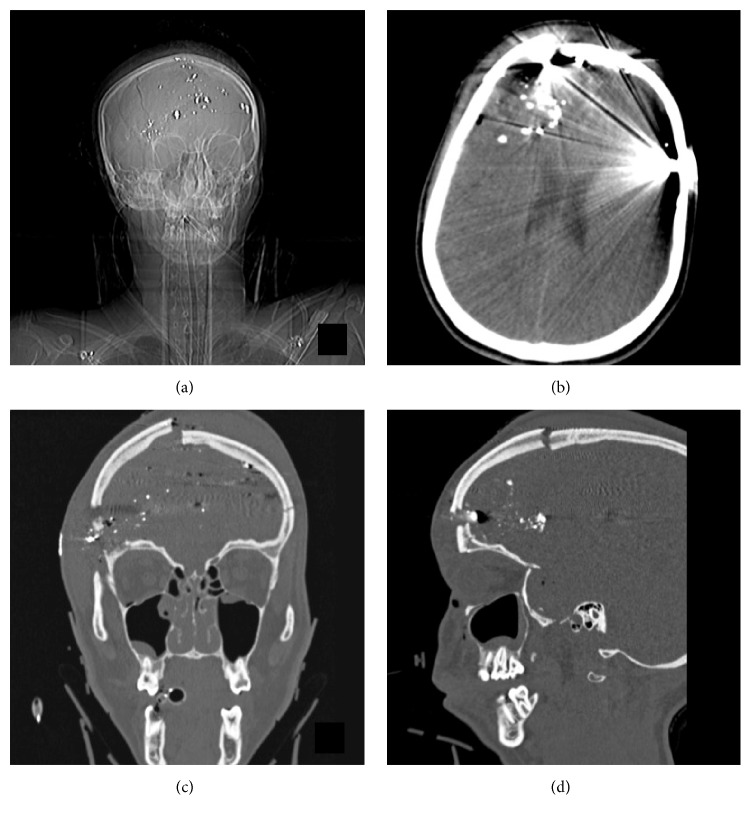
Preoperative computed tomography (CT) of the head and neck. (a) Scout image demonstrating multiple metallic fragments intracranially and in the bifrontal soft tissue and bone. (b) Axial noncontrast CT demonstrating an extensive right frontal skull fracture with intracranial acute blood products including intraparenchymal, subarachnoid, subdural, and epidural components. (c, d) Coronal and sagittal reconstructions demonstrating the entry and exit sites of the bullet and the comminuted displaced free floating right frontal bone fragment.
